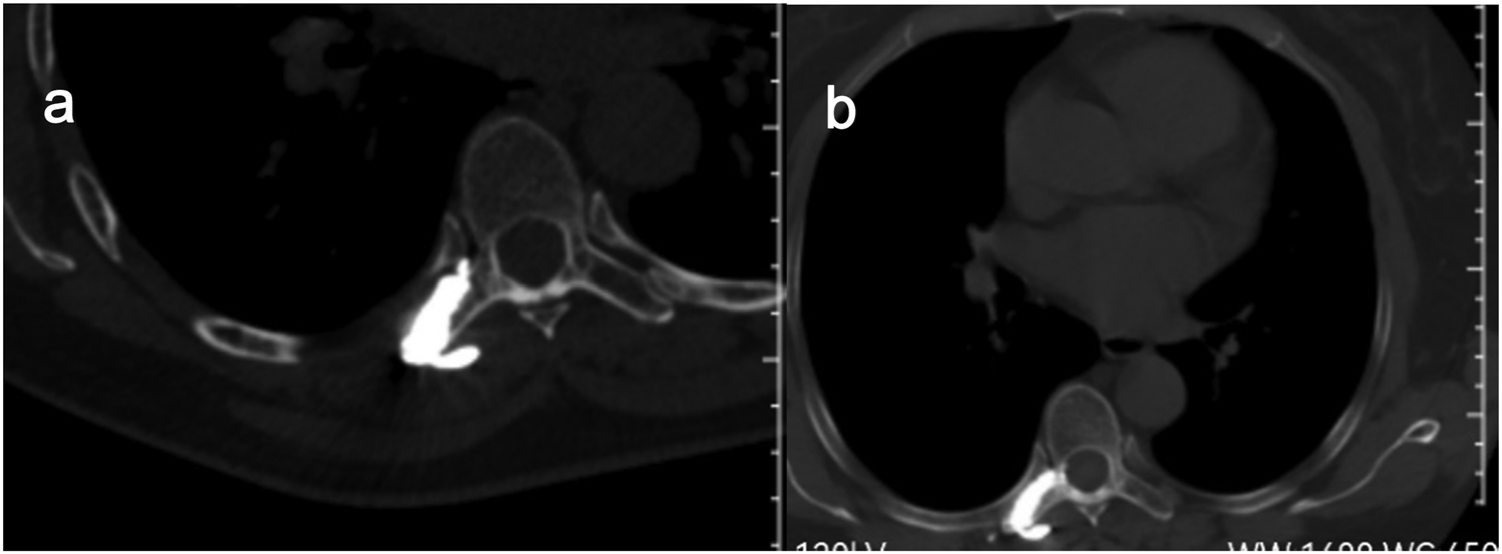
Postoperative CT showed intact cement filling and no leakage in the spinal canal (a) and follow-up after 6 months showed no displacement of the bone cement and no new bone destruction (b).
